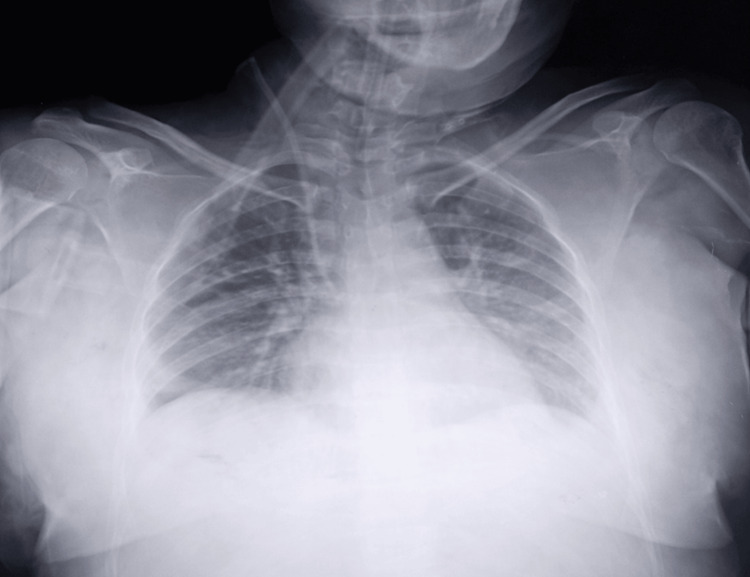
Chest radiograph taken shortly after ICU admission Although cardiomegaly is ideally assessed on a posteroanterior view, this portable anteroposterior film suggests an enlarged cardiac silhouette (estimated cardiothoracic ratio >0.5) with bilateral lower-zone air-space opacities consistent with pulmonary edema ICU: intensive care unit
